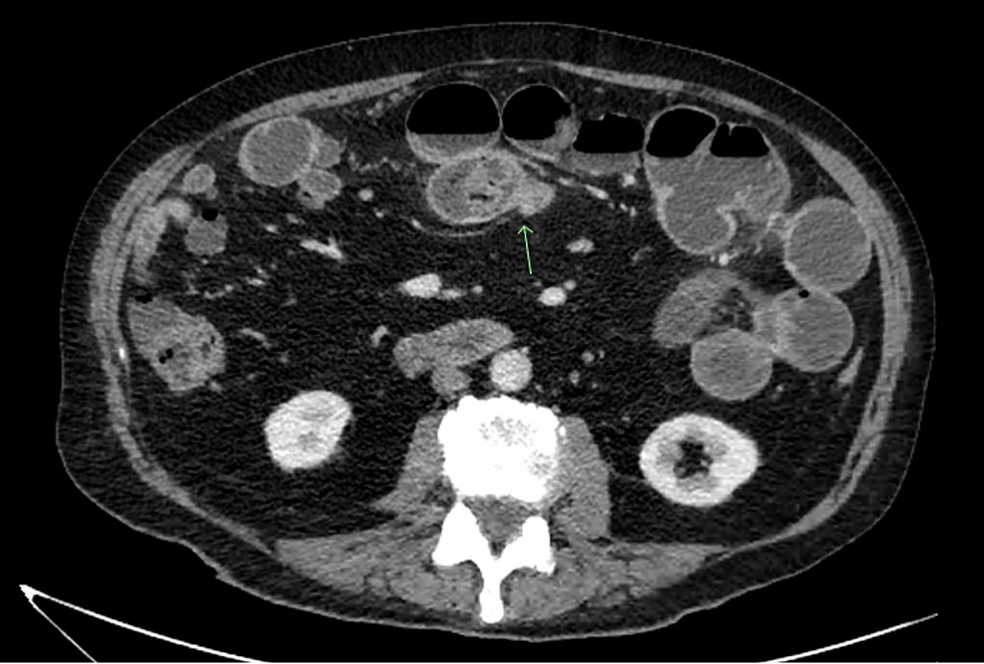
Contrast-enhanced CT scan demonstrating transition point of small bowel obstruction.
